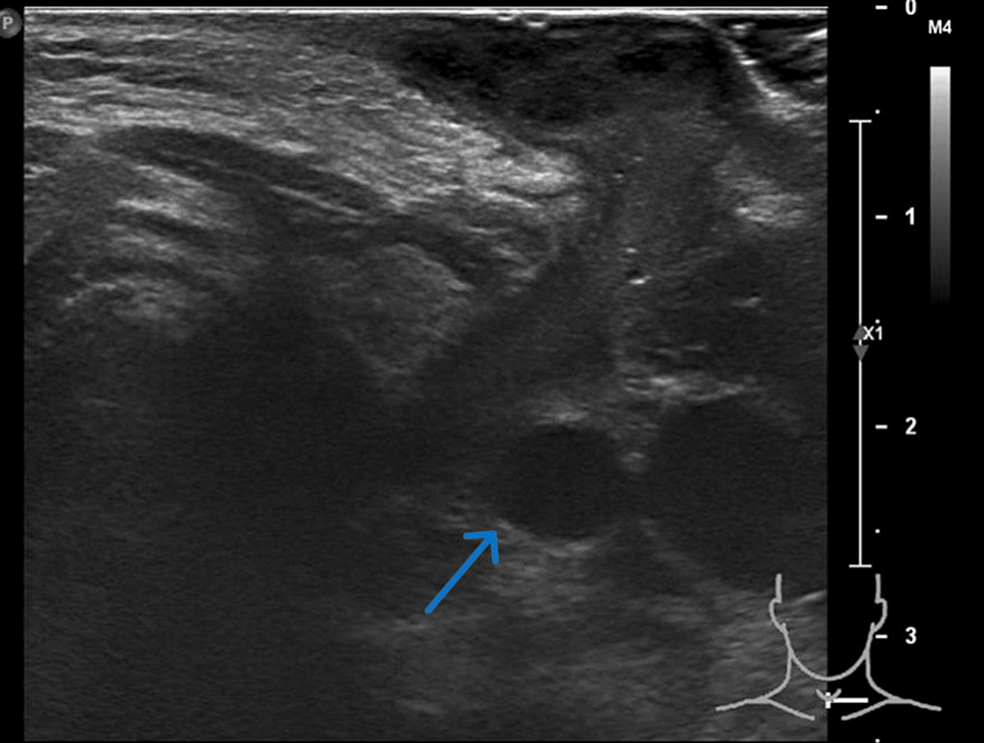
Ultrasonography image of the neck Ultrasound of the neck shows a collection within the left thyroid lobe (blue arrow).
